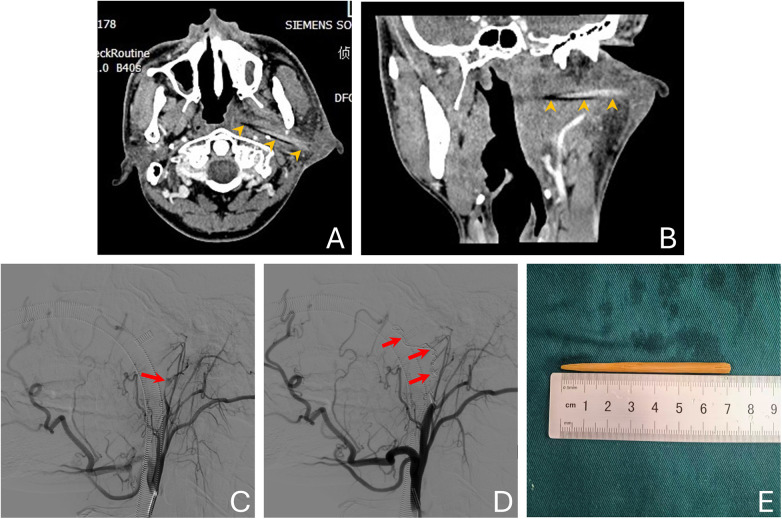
(A,B) Oropharynx CT scan showing significant swelling extending from below the left auricle to the left parapharyngeal space, with a strip-shaped high-density shadow inside (orange arrowhead) and associated pharyngeal stenosis. (C,D) Intraoperative facial angiography revealing local extravasation of the contrast agent (red arrow) from the maxillary artery (C), and several coils (red arrow) were used to perform maxillary artery embolization (D). (E) Image of the foreign body causing damage to the maxillary artery.
